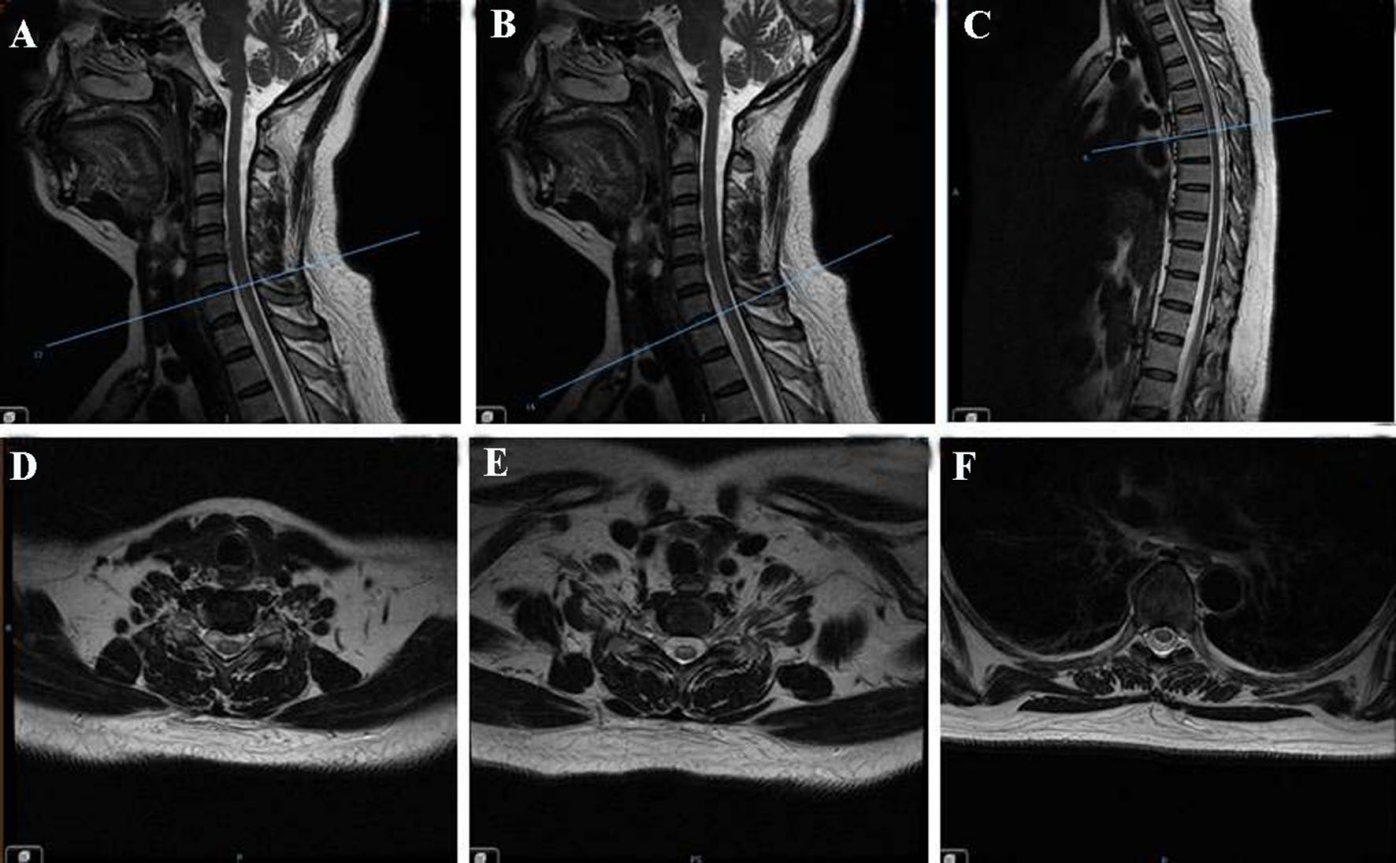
Magnetic resonance imaging (MRI) obtained one month of onset of the disease (sagittal section A–C; transverse section corresponding to the scaleplate, respectively D–F). The lesions were significantly absorbed compared with the anterior imaging
